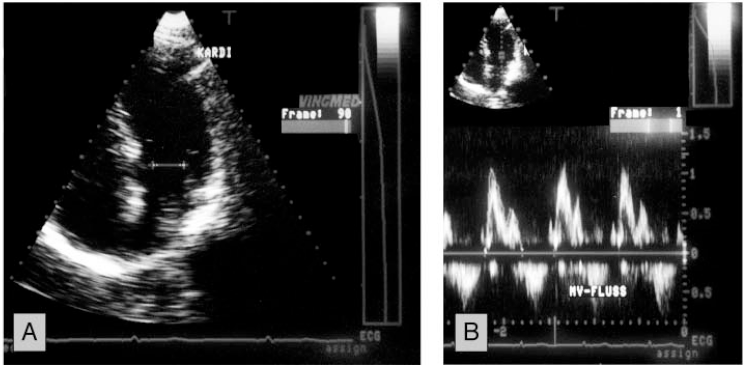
Example of the measurement of the flow area and the velocity time integral over the mitral valve. Panel A: The area of the mitral valve was measured from a diameter measured at the level between the mid and distal third of the valve leaflets in the apical 4-chamber view. Panel B: The VTI in the mitral valve was measured from the apical 4-chamber view.
